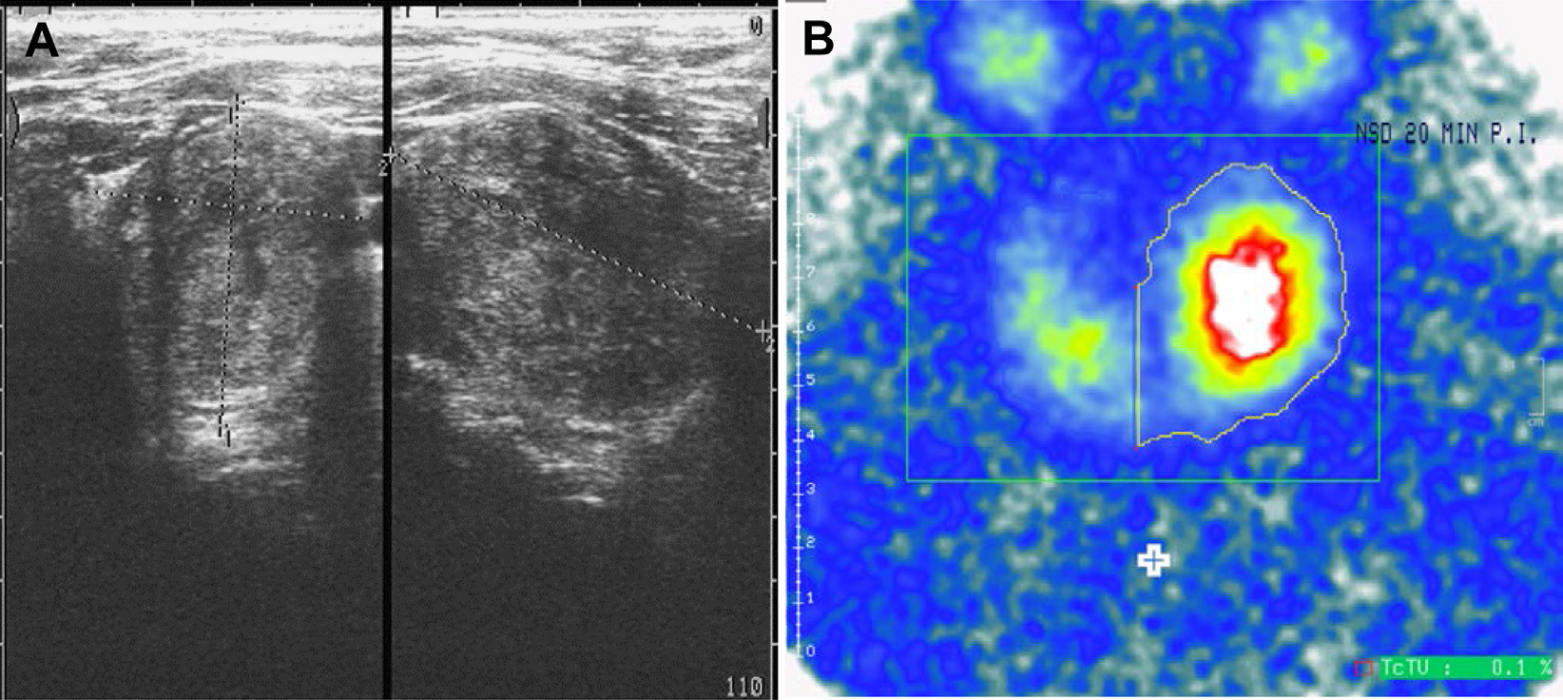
A, Sonographic finding of a nodule (approximately 2 × 3 × 4 cm) in the area of the left thyroid lobe. B, The nodule could be identified as parathyroid adenoma by technetium-99m sestamibi scintigraphy that shows intense tracer uptake in the area of lower left lobe of the thyroid. The other three parathyroid glands are colored green.
